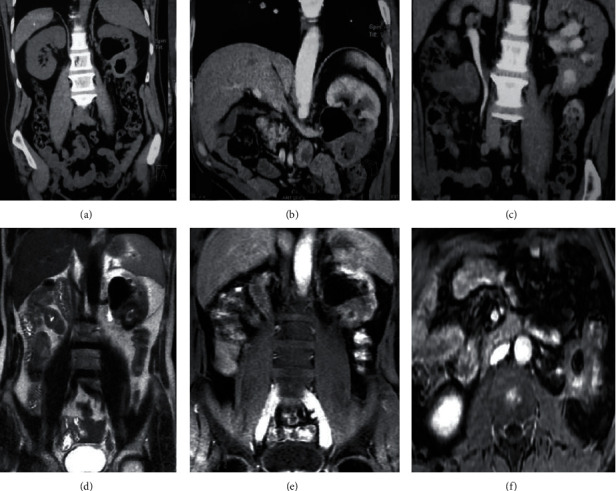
(a–c) Computed tomography; (a) pelvic hydroaeric level in the left kidney; (b) poorly enhanced lower pole in the left kidney; (c) contrast medium extending to the left colon. (d–f) Magnetic resonance imaging; (d) 41 × 38 × 41 mm T2 hypointense lower pole left renal mass associated with a pelvic hydroaeric level; (e) contrast medium extending to the left colon; (f) left renal mass invading the proximal ureter, the left colic angle, and the psoas muscle.
